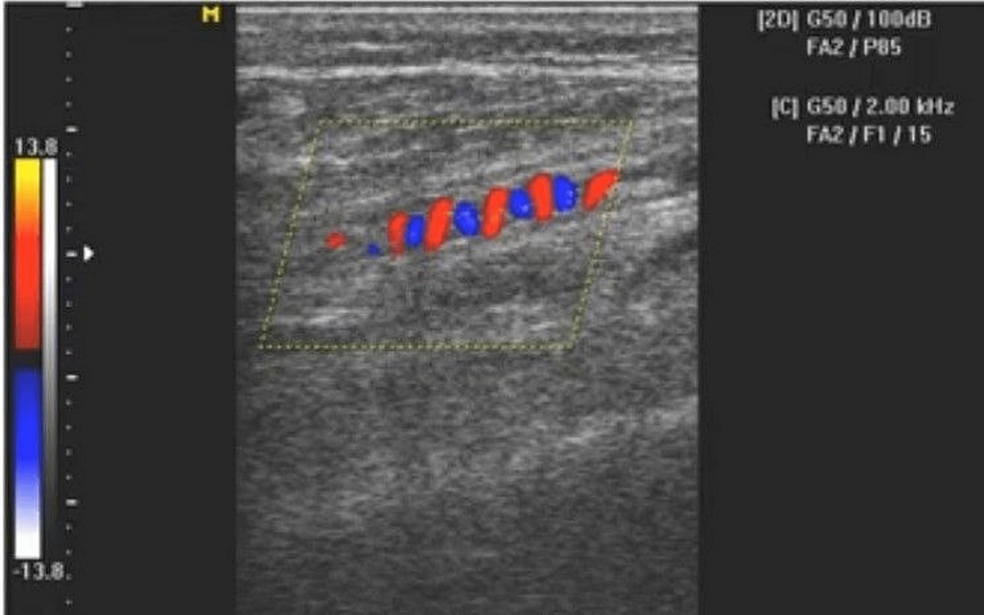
Doppler study image of lower limb showing distal small-sized corkscrew collateral vessels
